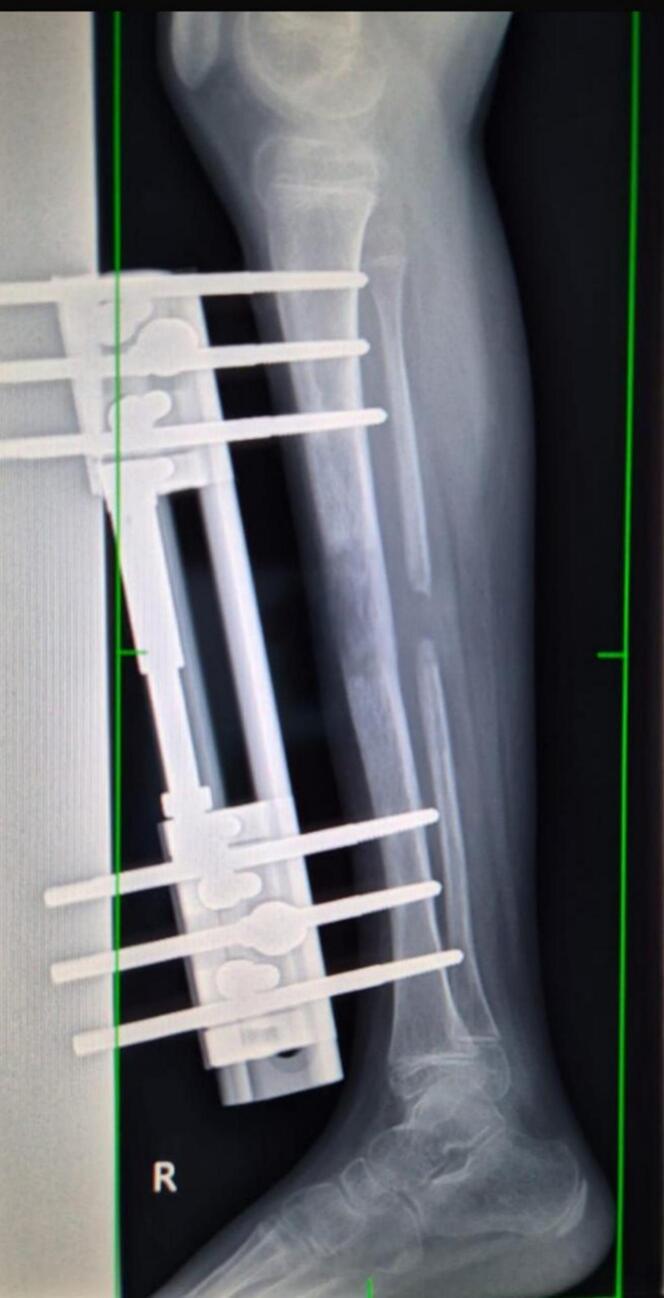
Xray Right leg (lateral view)- 6 weeks of distraction showing callus formation and bone lengthening of right tibia.
